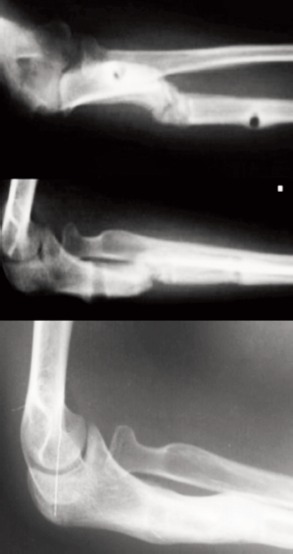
Radiograph at 3 months follow-up. Reduced radial head and osteotomy site united. At one year follow-up, radial head remain reduced.
